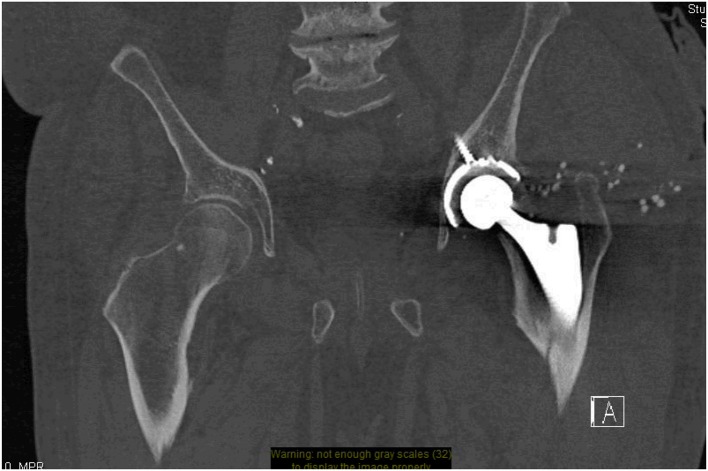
CT pelvis- minimally displaced left proximal femur peri-prosthetic fracture. Small round stimulant beads visible around the left side tracking down toward the left hip wound.
